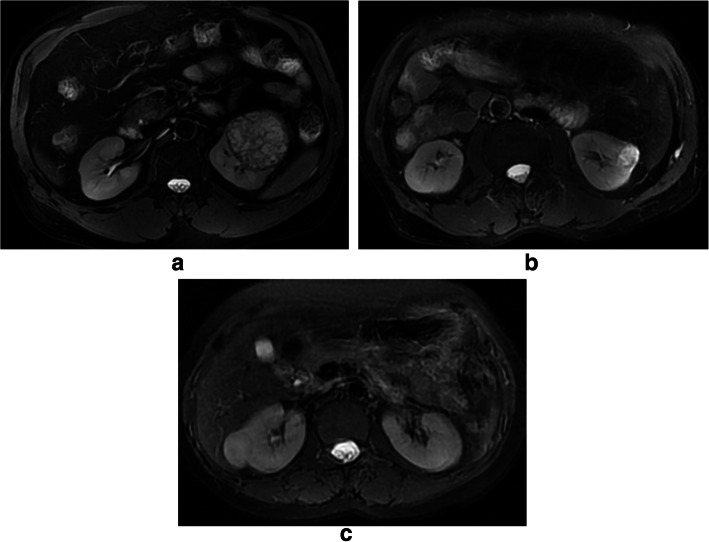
a-c: T2-weighted imaging signal intensity. CCRCC a and RO b showed hyperintense whereas AML c showed hypointense on T2-weighted imaging
